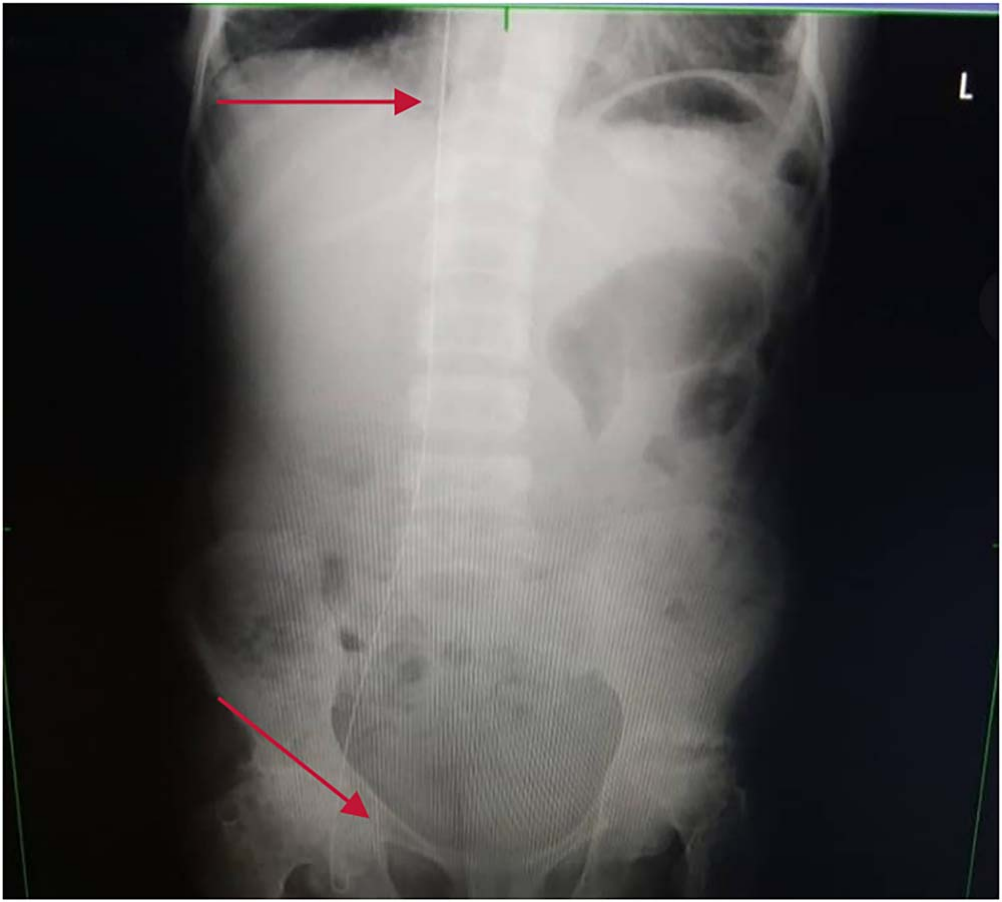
Plain abdominal X-ray extending from the IVC through the right iliac vein to the right femoral vein.
